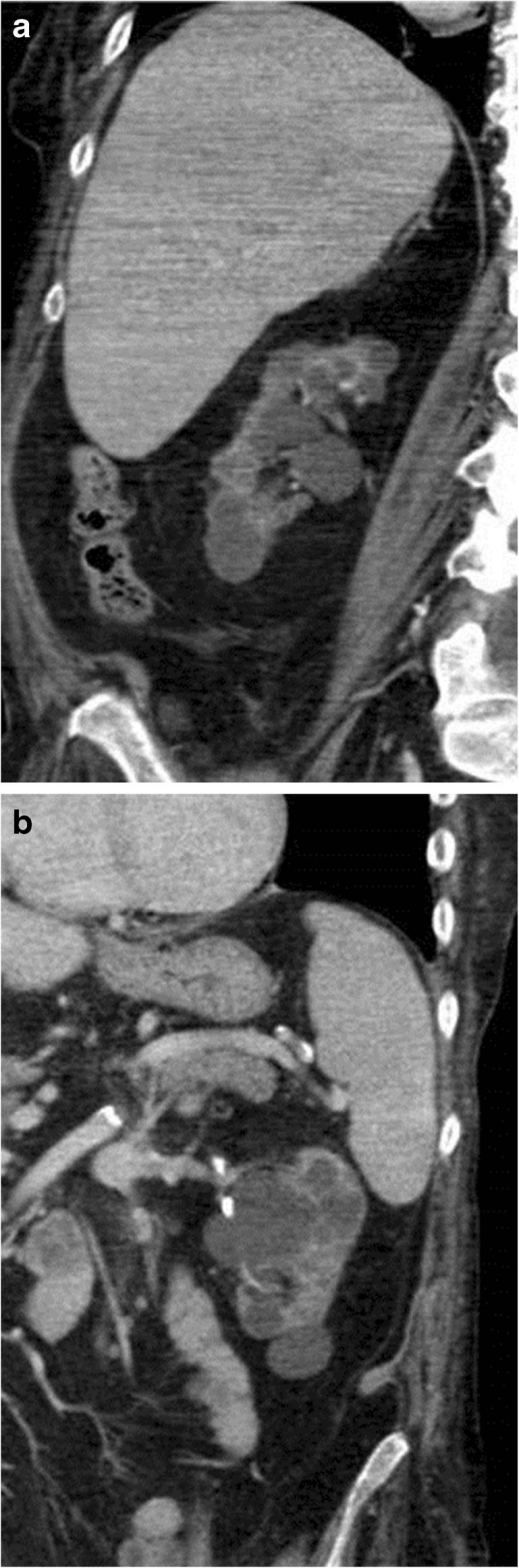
a, b: Unenhanced (a) and contrast-enhanced CT (b). Acquired cystic kidney disease in a patient undergoing long-term haemodialysis
